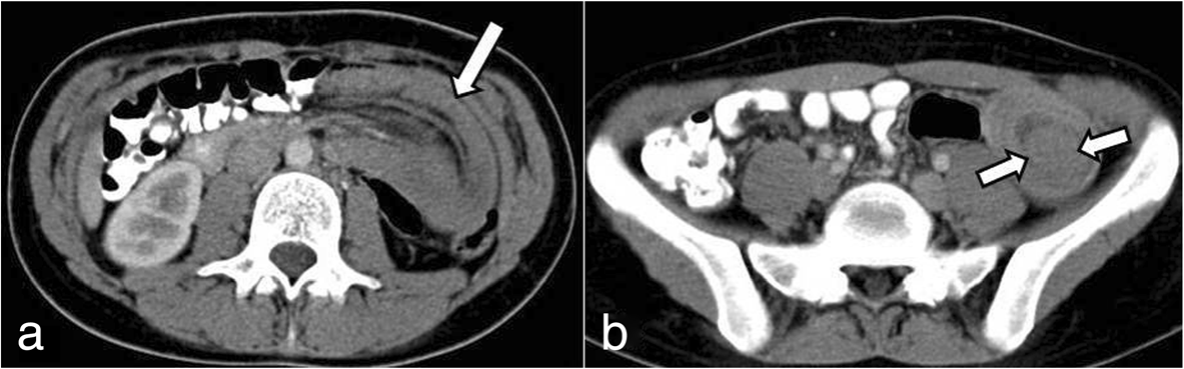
Abdominal computed tomography. (a) Small bowel intussusception (arrow). (b) A polyp within the intussuscepted loop (arrows).
